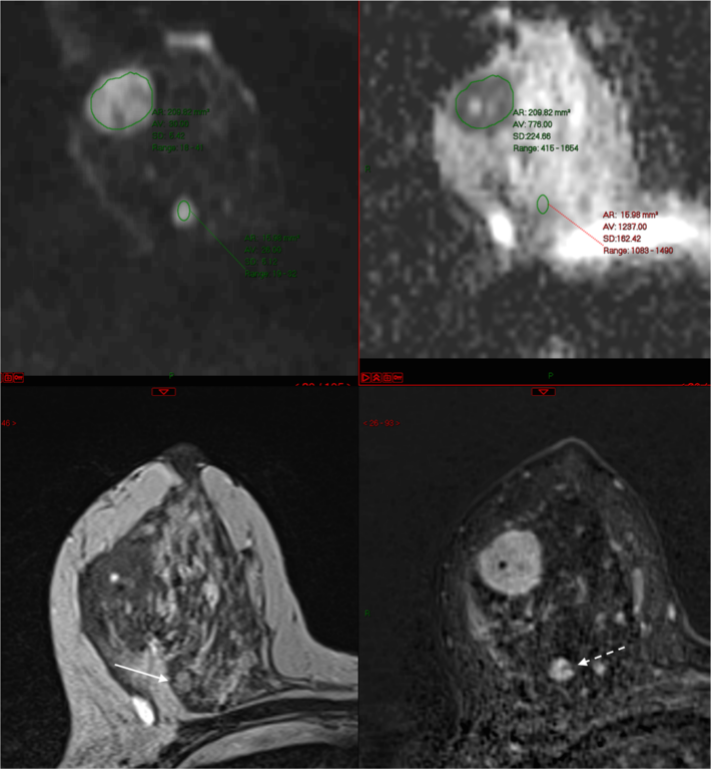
Multiparametric breast MRI. From top left to bottom right: DWI (b850), ADC map, T2W image and T1W post-contrast subtracted image. There is an obvious carcinoma in the upper outer quadrant of the right breast. An unexpected second rounded enhancing mass deep in the right breast is slightly hyperintense on the T2 weighted image (*arrow*) and has high signal on the b850 image, but there is no restriction of diffusion. Notice also a non-enhancing internal septation (*dashed arrow*). Biopsy-proven fibroadenoma
